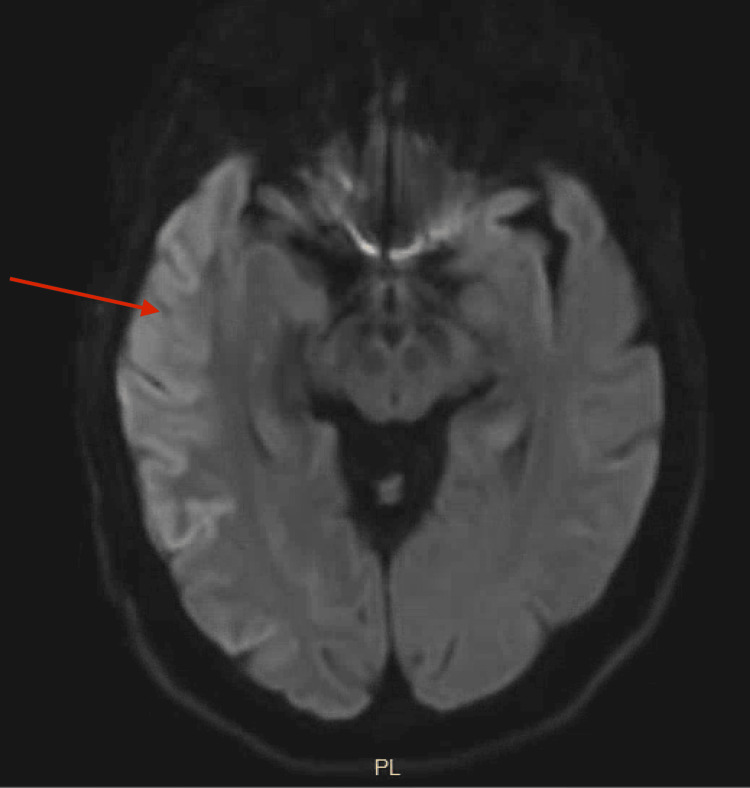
An MRI of the brain diffusion-weighted image showing hyperintensity in the right frontotemporal lobe
